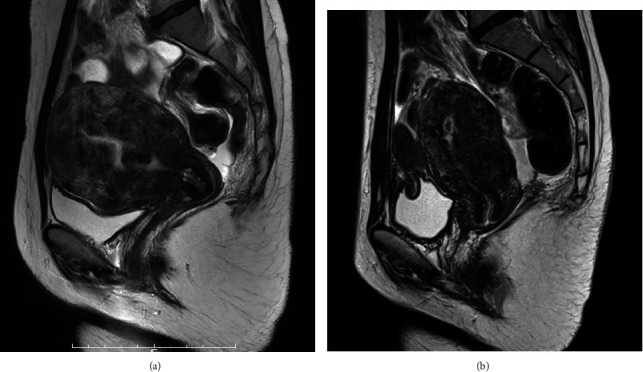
MRI T2-weighted findings of diffuse uterine leiomyomatosis (a) before and (b) after extensive myomectomy.
